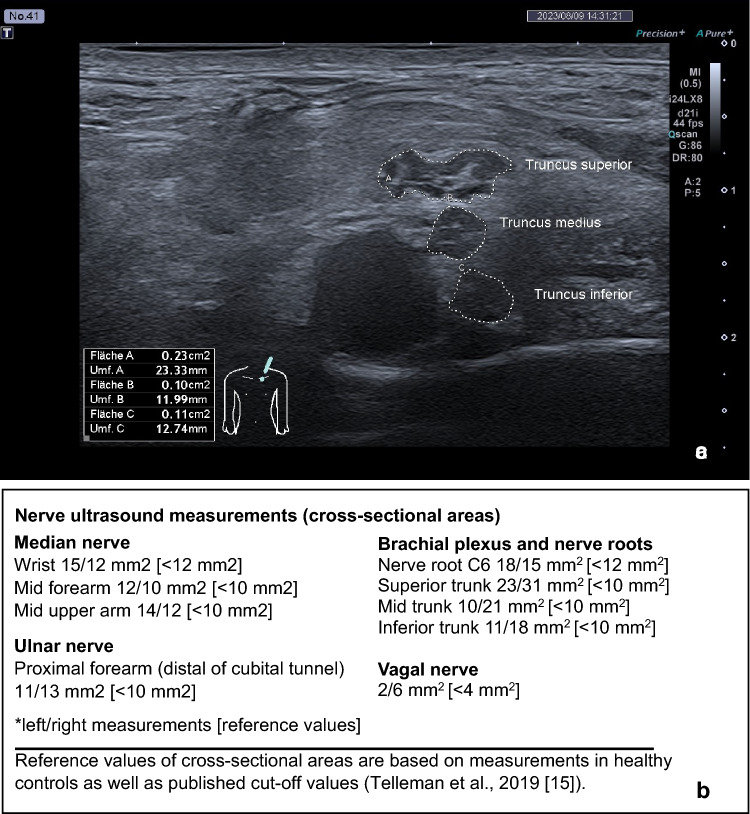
Nerve ultrasound examination. a Exemplary ultrasound image (Canon Applio i700; 21 MHz probe) of the left brachial plexus demonstrating enlargement of brachial plexus at the level of the trunks (Fläche = area; Umf. = Umfang = circumference). b Nerve ultrasound measurements [reference values in brackets]
